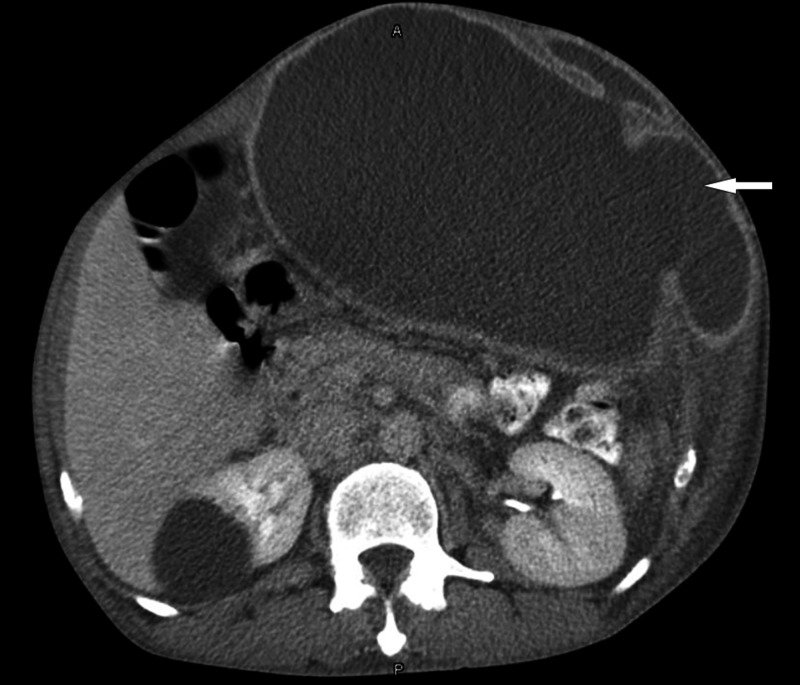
CT of the abdomen showing a left-sided rectus sheath abscess (arrow).
